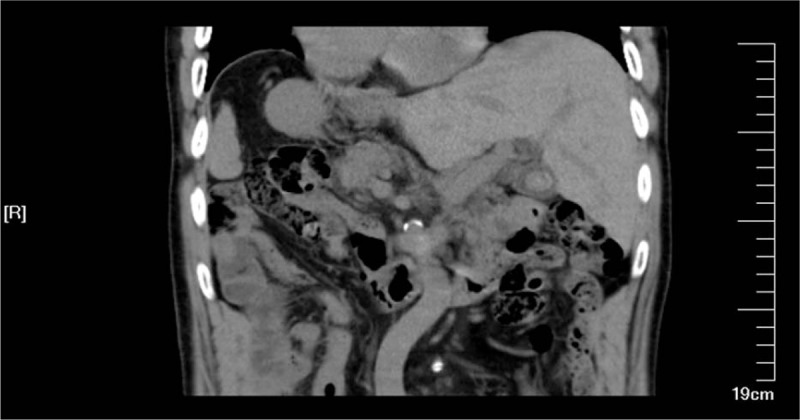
Computed tomography (CT) scan confirmed the diagnosis of situs inversus totalis (SIT) with gallbladder stone.
